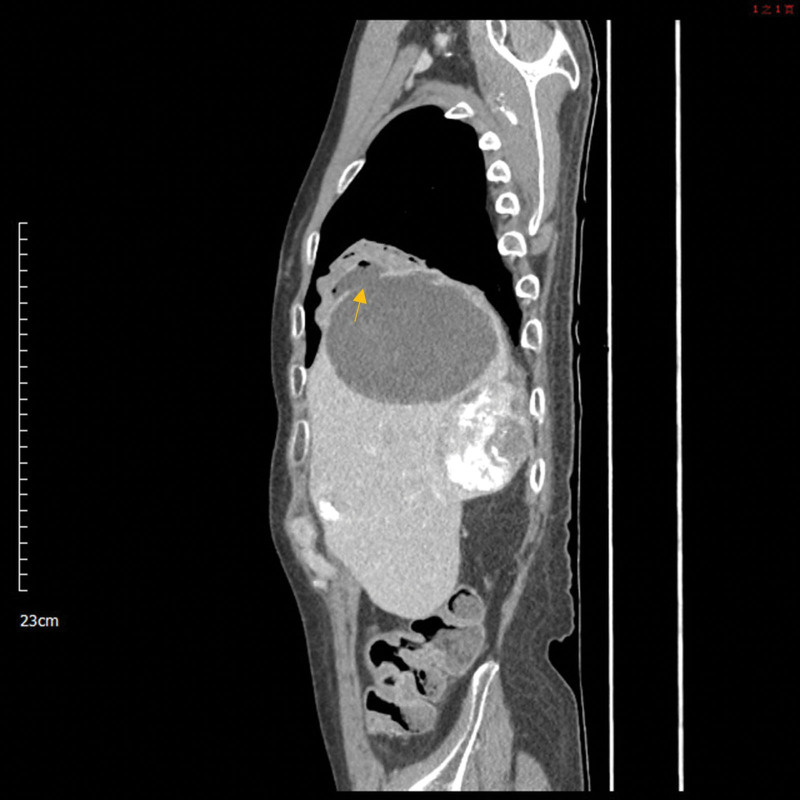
CT scan revealed a biloma and bronchial cyst with an air-fluid level connecting through a diaphragmatic defect (arrow). In addition, an inflammatory pulmonary consolidation in the lateral basal segment of the right lower lobe was also visible. CT = computed tomography.
